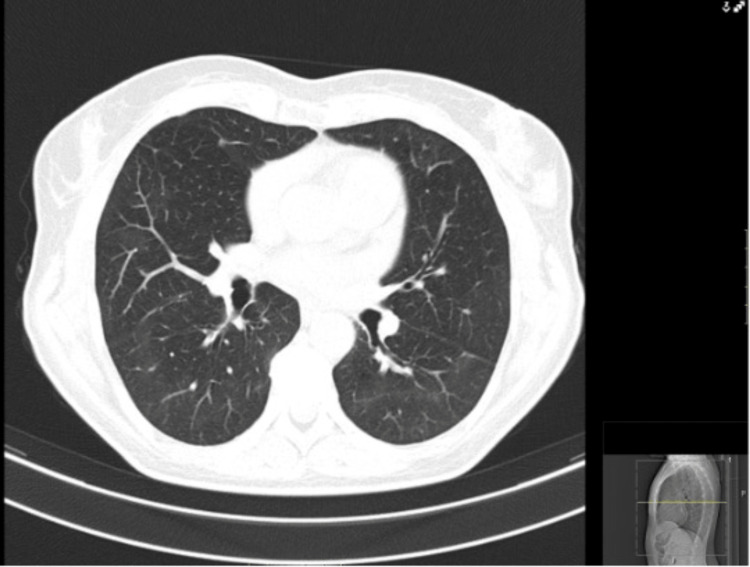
Follow-up chest CT scan, demonstrating a significant pulmonary improvement.
